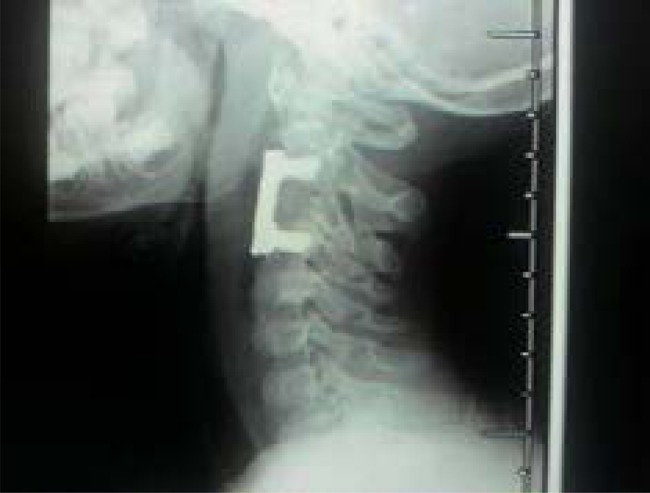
Post-operative lateral plain X-ray on the same patient after completion of the ACDF.
